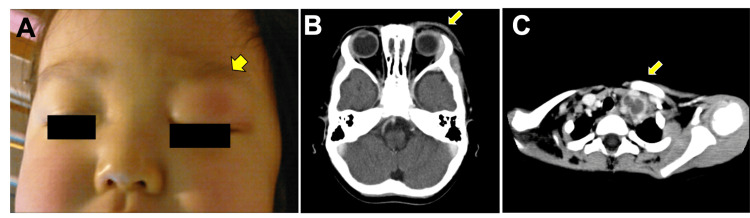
Clinical manifestations of the patient (A) Left supraorbital swelling in the patient. Arrowhead indicates the left supraorbital swelling and redness on admission. (B–C) Radiological findings of the patient. Enhanced computed tomography (CT) of the head and neck identified supraorbital skin thickening with irregular subcutaneous fatty tissue concentration (B) and an enlarged left supraclavicular lymph node (C).
